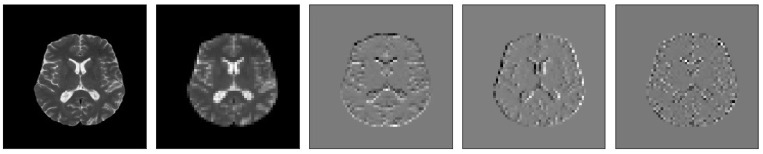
Samples of our Level 2 DWT decomposition of a healthy MR image type T2.
